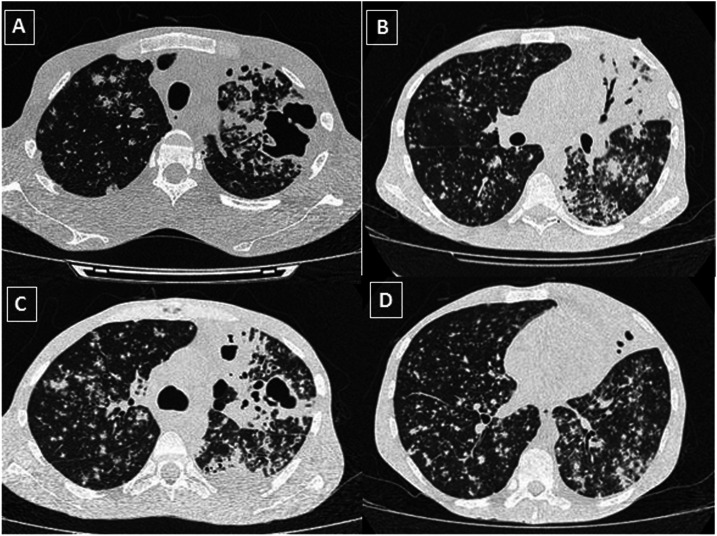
Chest computed tomography (CT). (A–C) Axial thin-section unenhanced CT image revealing a large cavitation with air bronchograms and consolidation. (B–D) Axial thin-section unenhanced CT image showing randomly distributed (miliary) nodules in both lungs associated with glass-ground opacities and consolidation in the left lower lobe.
